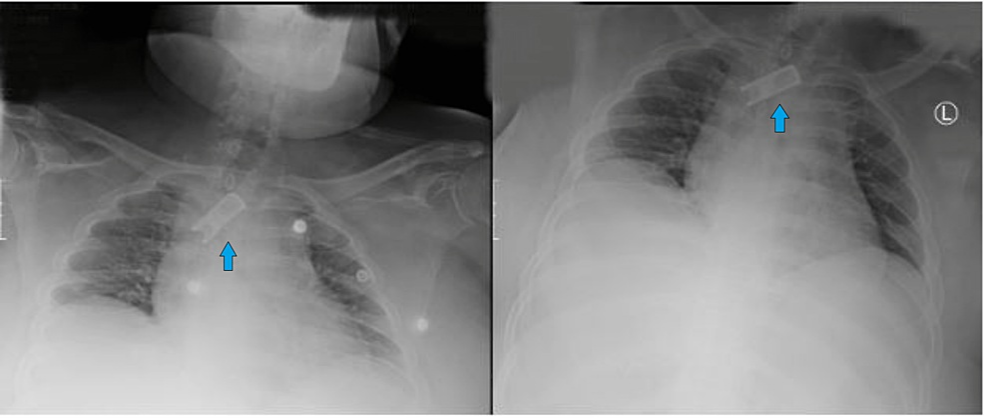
Pre-endoscopy X-ray image showing the foreign body
